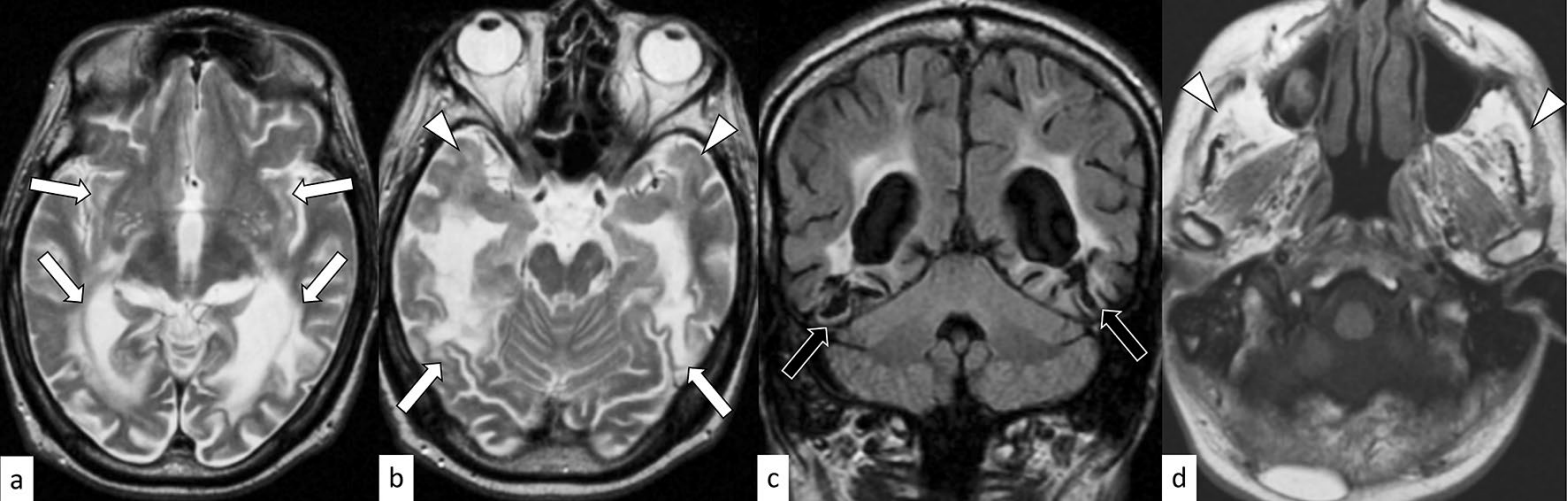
A 56-year-old man with DM1. T2-weighted axial images (a, b) show hyperintensity with atrophy in the white matter of the bilateral subcortical insular and temporal lobes (arrows). Subcortical white matter hyperintensity is also found in the bilateral temporal poles (b, white arrowheads). Part of the T2 hyperintensity areas shows cystic changes on FLAIR coronal image (c, black arrows). Severe atrophy of the bilateral temporalis and masseter muscles with fat replacement is observed on T1-weighted axial image (d, arrowheads)
